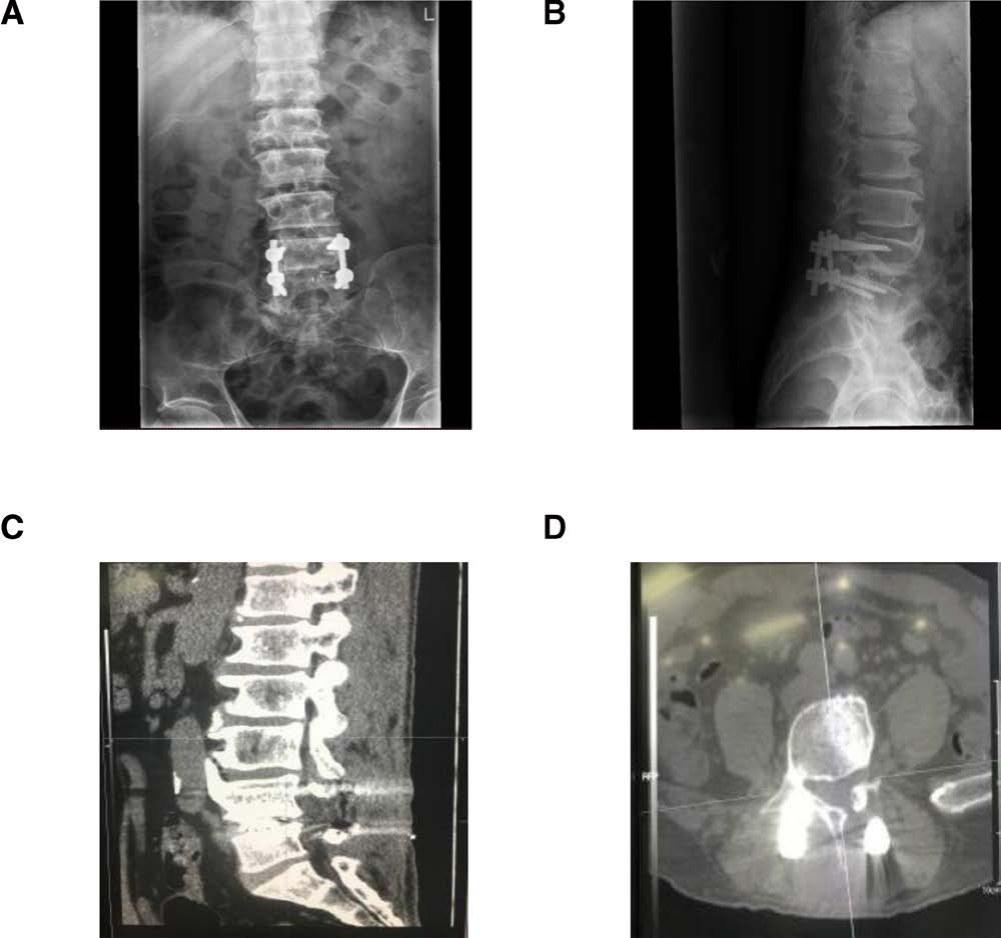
Postoperative X-ray and computed tomography scan. (A) Anterior-posterior X-ray view; (B) lateral X-ray view; (C) median sagittal section; (D) transverse section. Postoperative computed tomography scan showed complete decompression of the L4-5 level of the spinal canal.
